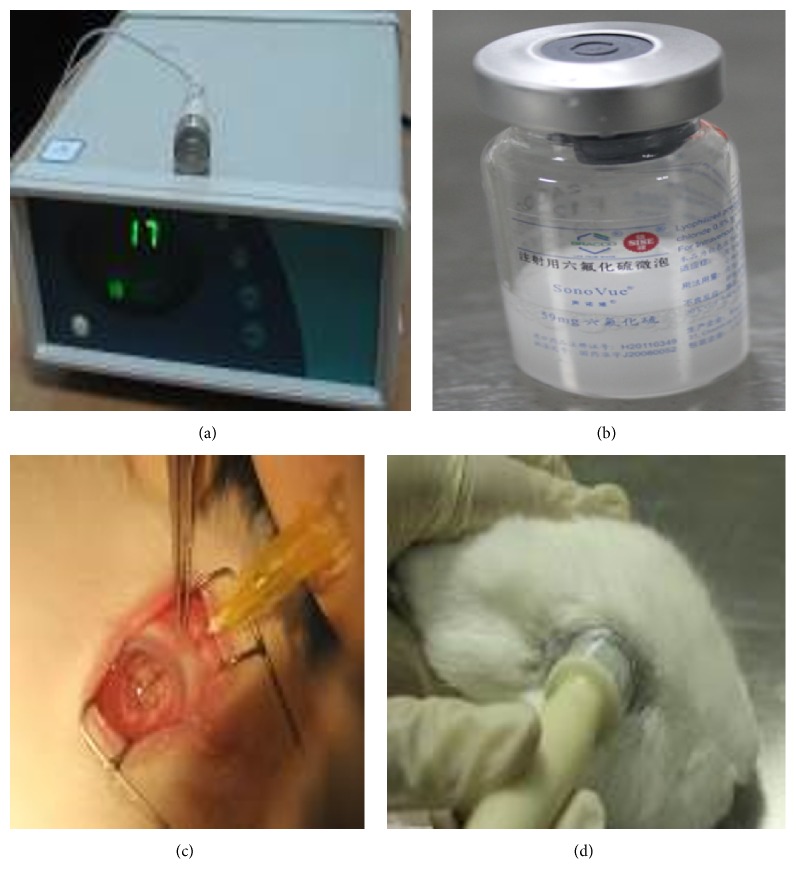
Preparation of ultrasound microbubble. (a) Ultrasonic instrument. (b) SonoVue. (c) mNGF injected into the vitreous. (d) Ultrasound irradiation on the rabbit's eye.
